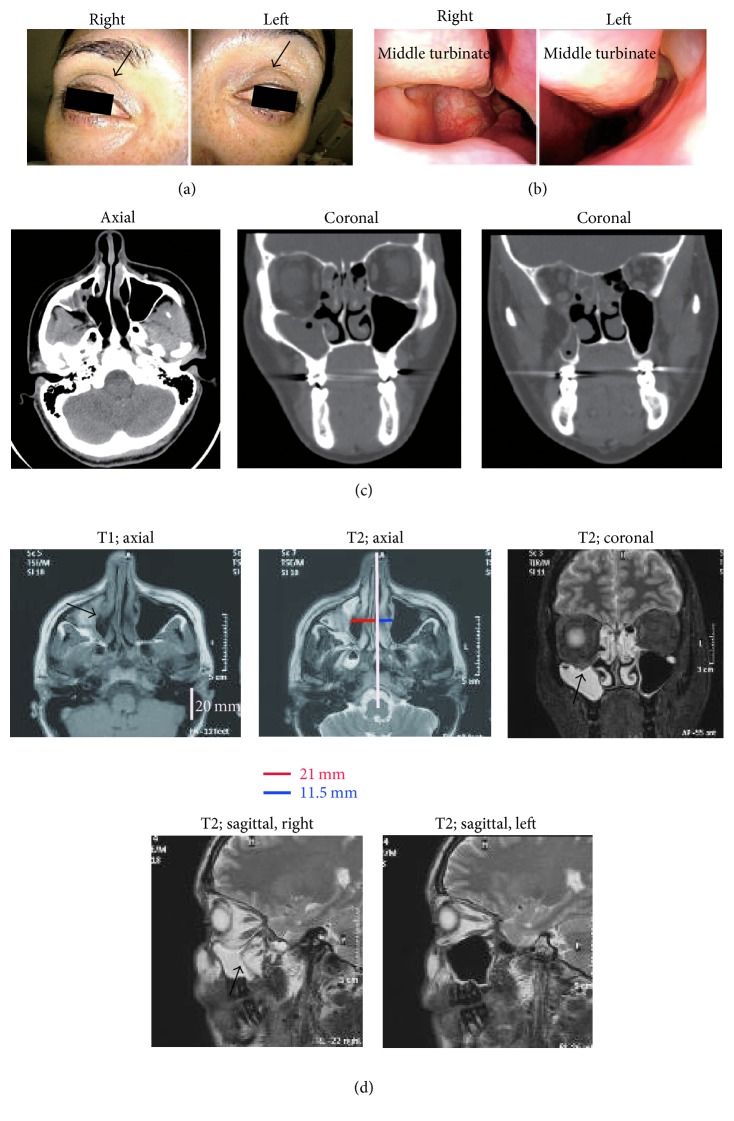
Summary of the clinical examination at the time the patient presented at our hospital. (a) Facial appearance: the arrows in the right and left pictures indicate the superior sulcus. The right superior sulcus appears to be deeper than the left superior sulcus. (b) Endoscopic findings: the right middle meatus is more enlarged than the left middle meatus. (c) Axial and coronal views on computed tomography. (d) T1 and T2 weighted images on magnetic resonance imaging. The T1 axial image shows that the right medial wall of the maxillary sinus (shown by the arrow) deviates laterally. The T2 coronal image indicates inferior bowing of the inferior wall of the orbit (shown by the arrow). The T2 sagittal images show prominent deviation of the posterior wall in the right maxillary sinus (shown by the arrow in the right image) relative to the structure in the left maxillary sinus (left image). The red and blue lines in the T2 axial image indicate the distance from the center line to the most deviated medial wall of the maxillary sinus.
